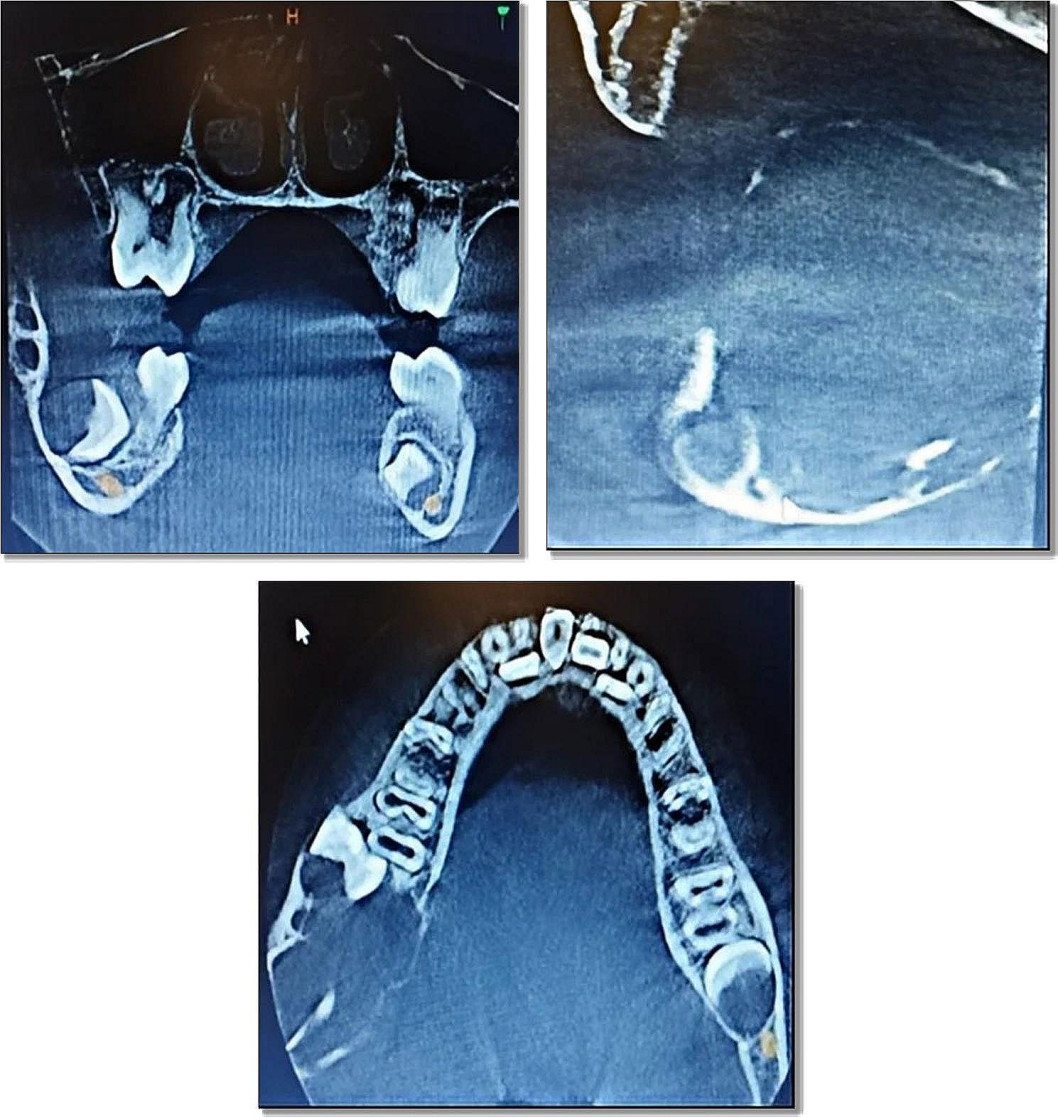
CT scan showing the extension of lesion all three planes ; Note that the lesion has eroded the cortex and is expanding into the soft tissue
